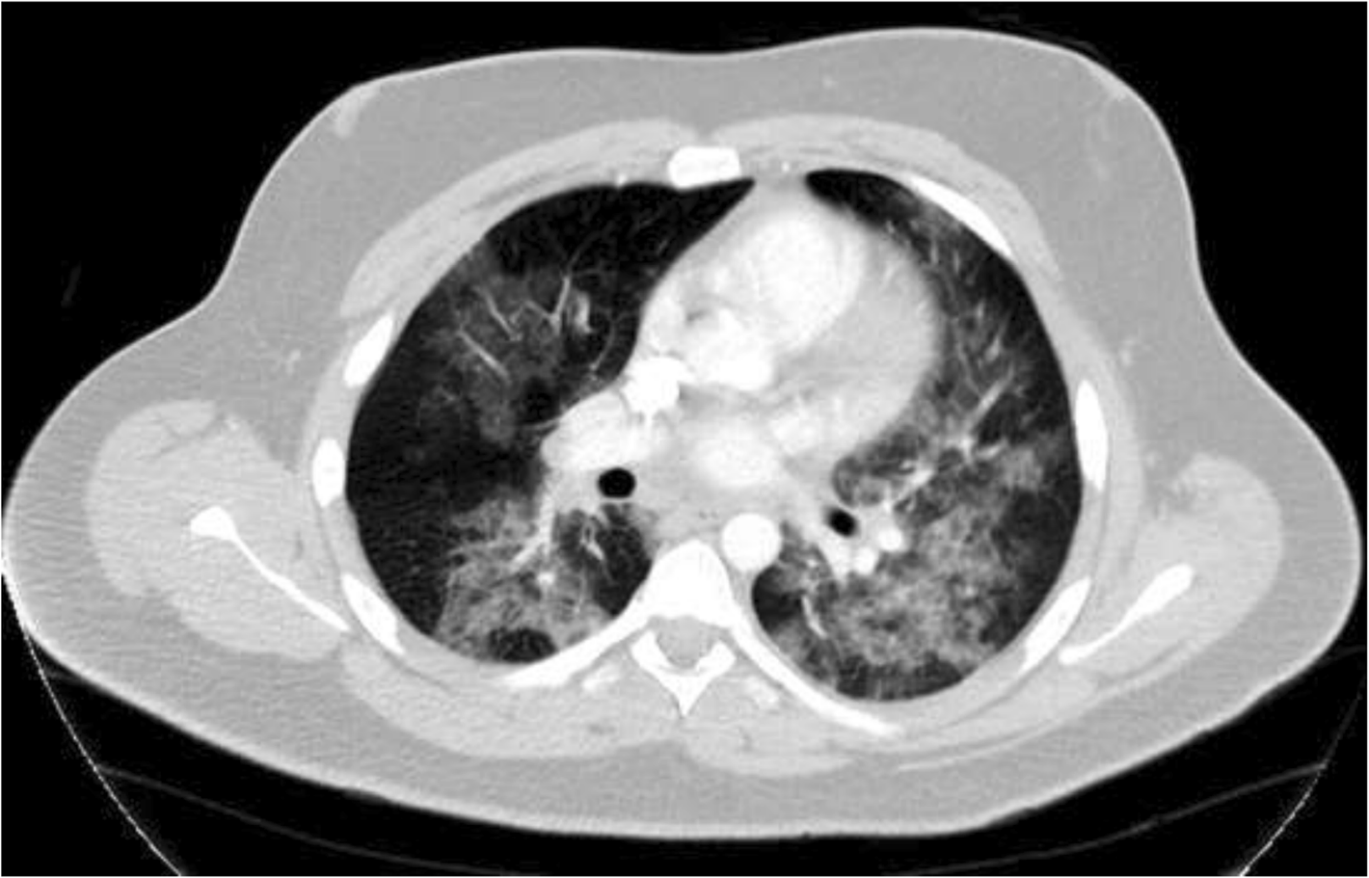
Axial CT Chest Scan with IV contrast: Diffuse bilateral ground-glass opacities throughout both lungs, demonstrating predominantly a perihilar distribution, with areas of mild bronchial wall thickening. Several mildly prominent hilar lymph nodes, the largest measuring 1.2 cm in the short axis on the right
